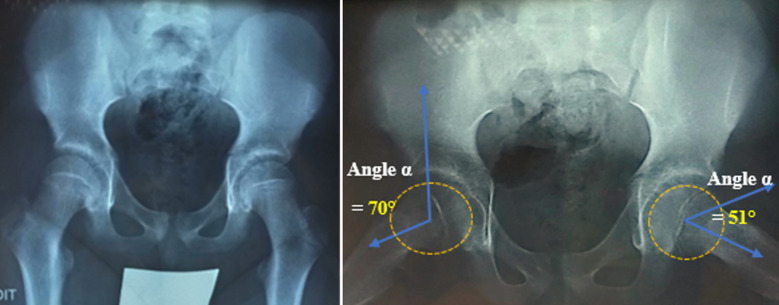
radiographies de face et de profil des hanches montrant un déplacement de la tête fémorale droite
